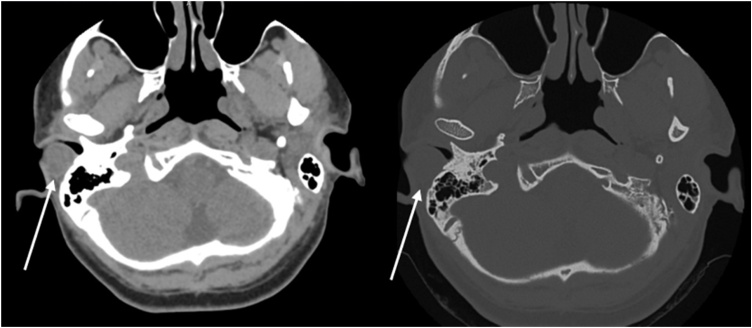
CT scan shows a lesion (arrow) along the posterior wall of the right external auditory canal, in contact with the mastoid.
